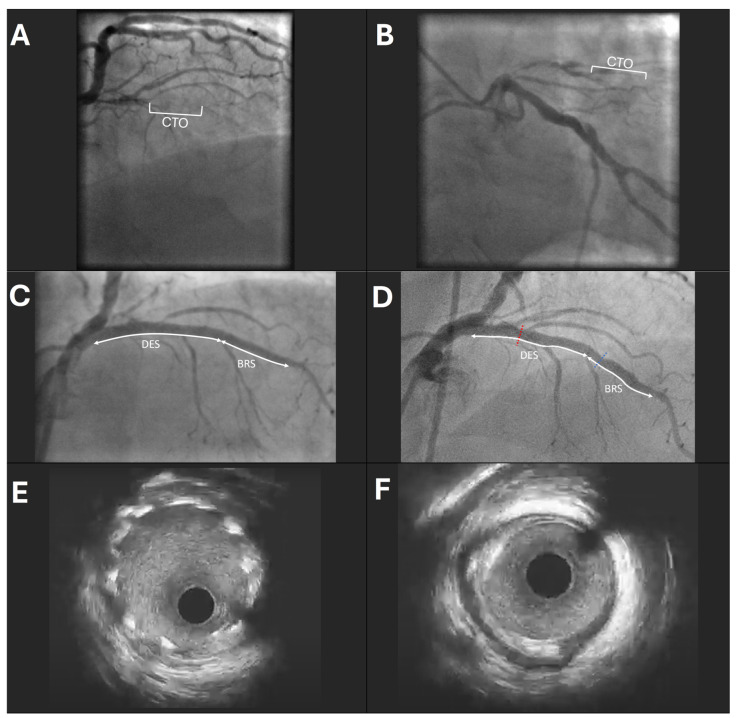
Representative case example of a patient with chronic total occlusion (CTO) in the left anterior descending artery (LAD) (A,B); (C) result of index percutaneous coronary intervention after implantation of 2 drug-eluting stents (DESs) and 1 bioresorbable scaffold (BRS) in a total length of 55 mm; (D) follow–up after six years; the patient is free from any major adverse cardiac events; the red dotted line (in D) represents the intravascular ultrasound (IVUS) cross-section of the DES without significant neointimal hyperplasia (E); the blue dotted line (in D) represents the IVUS cross-section of the BRS where the scaffold is fully dissolved (F).
